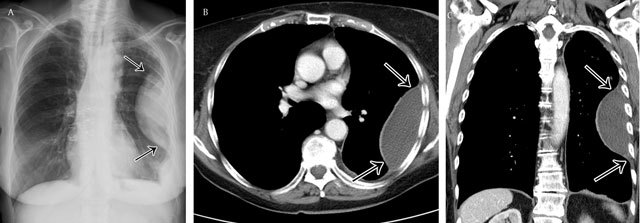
Pleural opacity. Encapsulated pleural effusion – Frontal chest radiograph (a) shows a lenticular opacity with smooth borders and obtuse angles (black arrows). Corresponding axial (b) and coronal (c) reformatted images demonstrate clearly the encapsulated pleural effusion.
